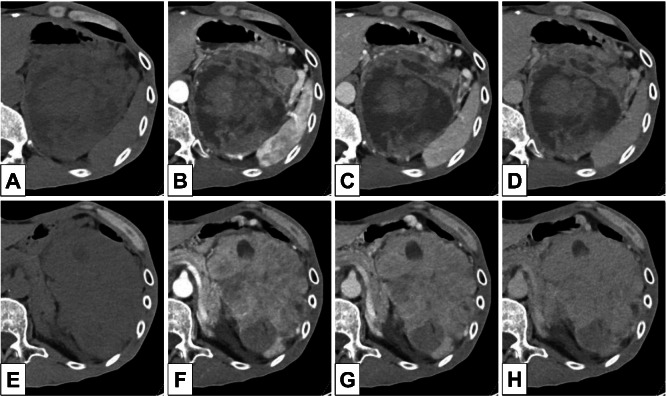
Contrast enhanced dynamic CT images. A well-defined tumor is present in the left upper abdomen. (A) Non-contrast CT of the upper portion of the tumor. (B) Arterial phase of the upper portion of the tumor. (C) Portal venous phase of the upper portion of the tumor. (D) Venous phase of the upper portion of the tumor. (E) Non-contrast CT of the lower portion of the tumor. (F) Arterial phase of the lower portion of the tumor. (G) Portal venous phase of the lower portion of the tumor. (H) Venous phase of the lower portion of the tumor. The upper portion of the tumor predominantly contains areas of fluid density, which do not enhance. The lower portion predominantly contains soft tissue density, which enhances in the arterial phase and shows diminished enhancement in the venous phase.
